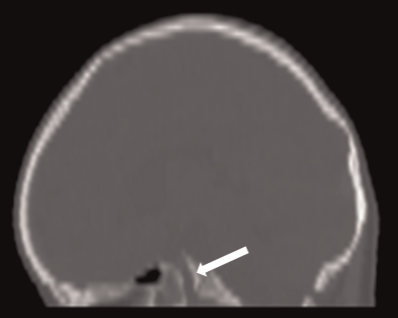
CT scan of the head with sagittal reformat on bone window through the midline shows defect (arrow) in the sphenoid body.
